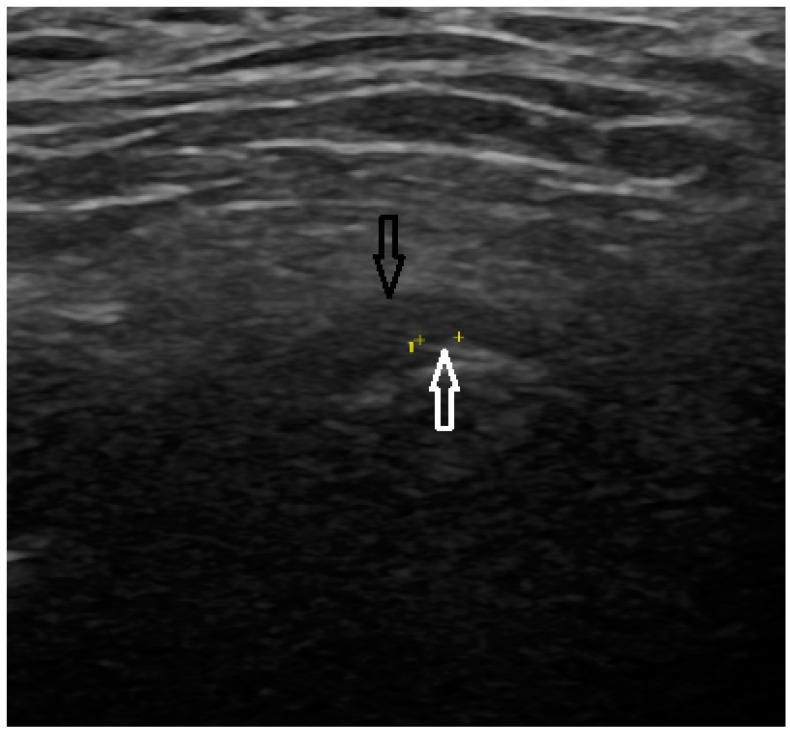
Ultrasound (articular disc—black arrow, osteophyte—white arrow).
